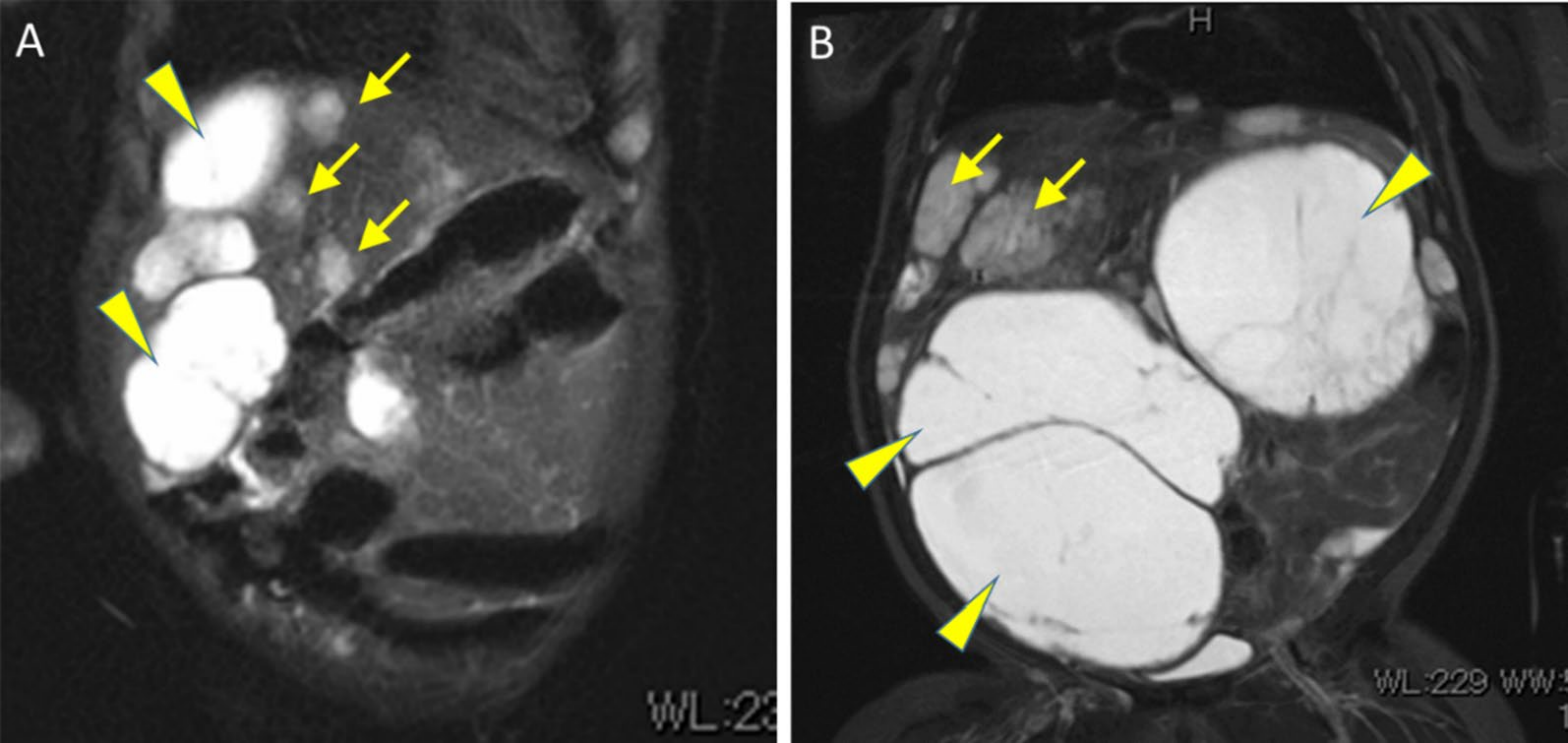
Coronal T2-weighted MR image at 2 months (A) and at 9 months (B). Both cystic (arrowheads) and solid (arrows) components show rapid increases after 7 months
